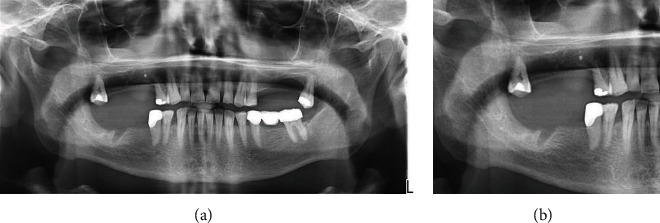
(a, b) Final OPG X-ray (17/06/2021): no relevant findings on the extraction sites (magnification (b)).
